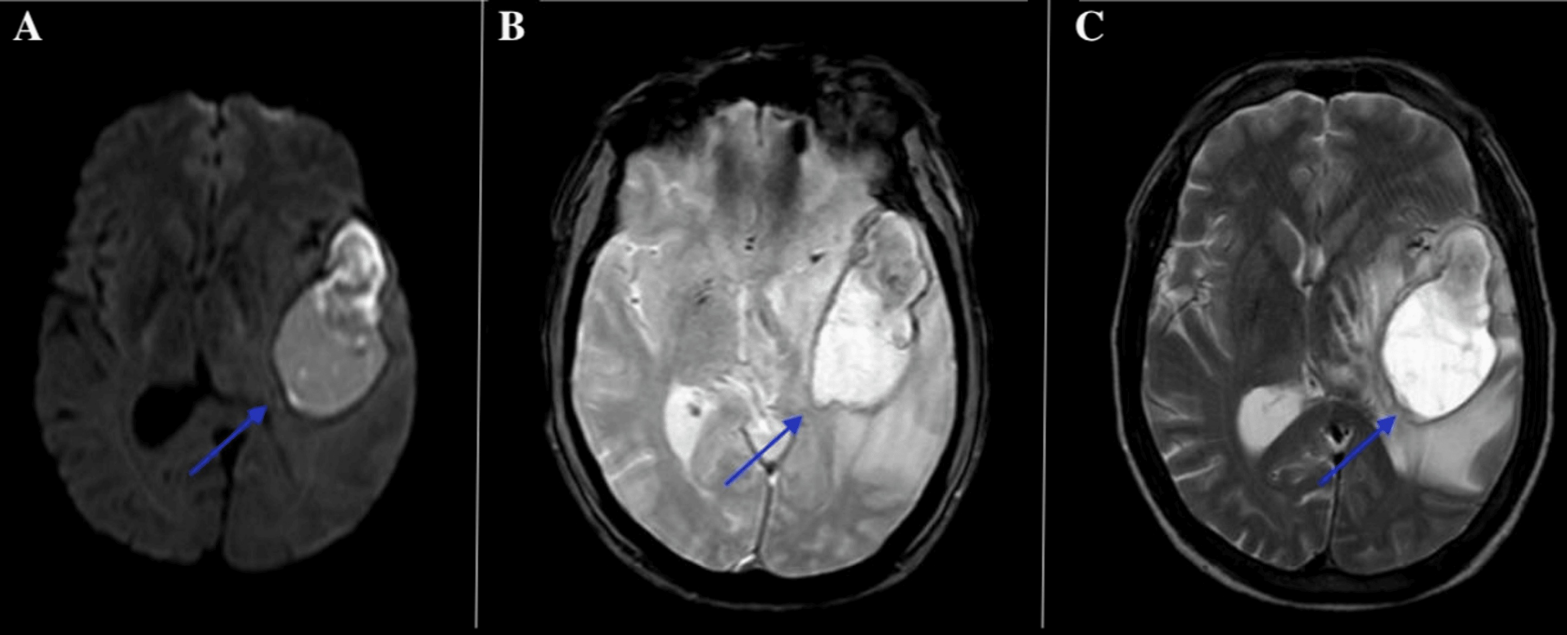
MRI brain images (axial sections) showing (A) diffusion-weighted imaging, (B) susceptibility-weighted imaging, and (C) T2-weighted imaging. The blue arrows indicate a well-defined hyperintense lesion involving the right thalamus and adjacent structures, consistent with an acute hemorrhagic lesion.
